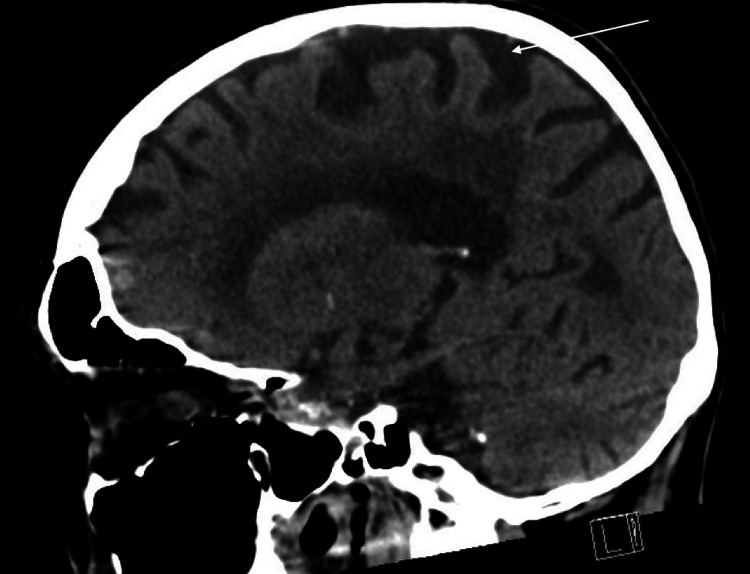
CT brain without contrast, sagittal view. Diffuse cerebral volume loss noted.
